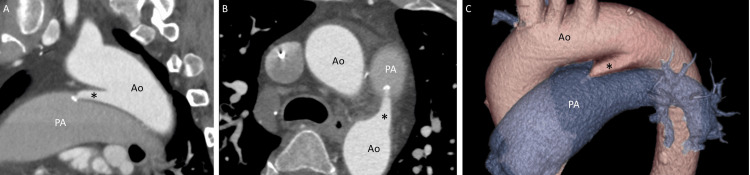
Computed tomography. A direct connection (asterisks) between the aorta (Ao) and the pulmonary artery (PA) is observed, with a small calcification at its exit to the PA, as seen in the sagittal image (Panel A), short-axis image (Panel B), and three-dimensional reconstruction (Panel C).
